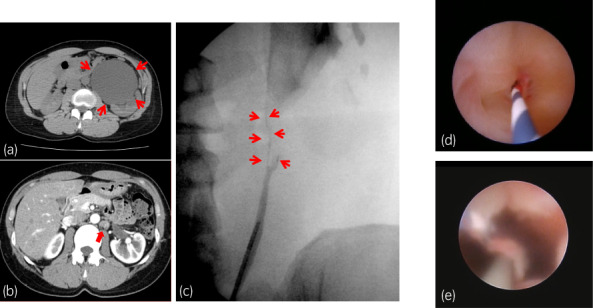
(a) CT shows hydronephrosis (red arrowheads) without crossing vessels when the patient presented with worsening left backache. (b) Contrast‐enhanced CT shows the thickened wall of the UPJ and an increase in the concentration of surrounding fat (arrowheads). (c): Retrograde pyelography when anatomic stenosis occurred showing the stenosis length (white arrowheads). (d) Ureteroscopy shows ureteral stenosis. (e) Ureteroscopy shows improvement of ureteral stenosis after redo pyeloplasty.
